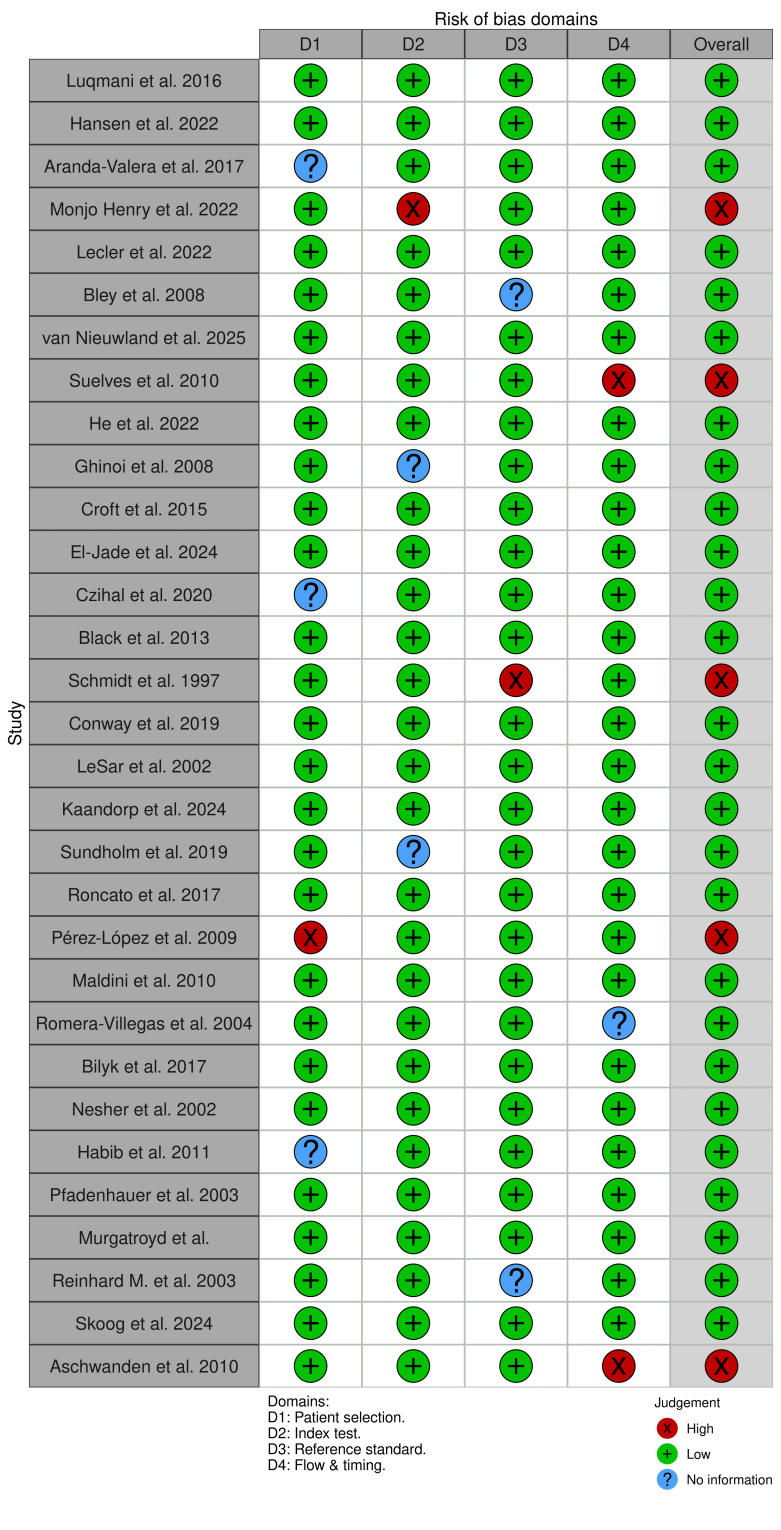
Ultrasound cohort QUADAS-2 results QUADAS 2: Quality Assessment of Diagnostic Accuracy Studies 2 tool. [13-43]
